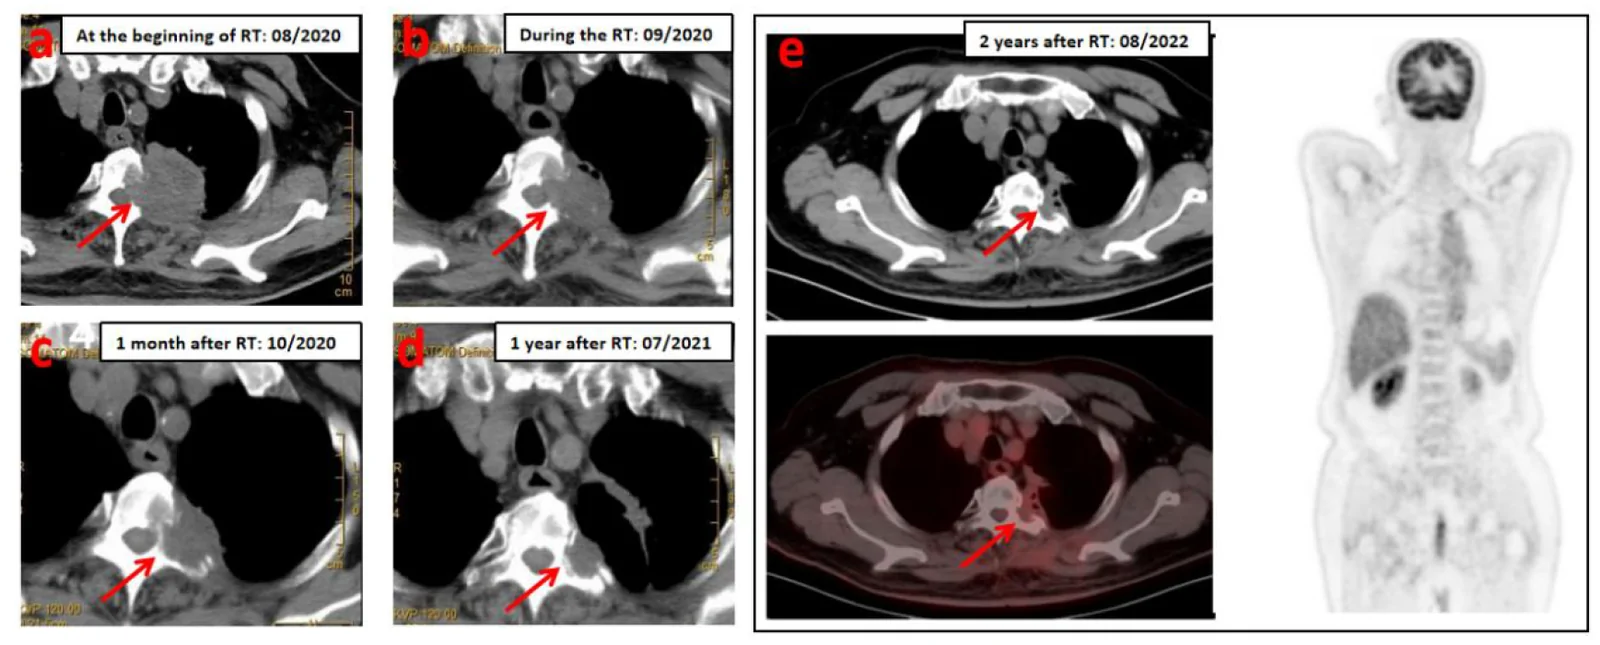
Dynamic changes of tumor-invaded vertebrae bone after radiotherapy. There is extensive destruction of the tumor-invaded vertebrae at the beginning of (a), during (b), 1 month (c) and 1 year (d) after radiotherapy (RT) and slow but gradual shrinkage of the destroyed bone to almost normal appearance at 2 years (e). At two years after RT, the PET-CT shows minimal residual tumor, without remarkable metabolic activity of the tumor.
